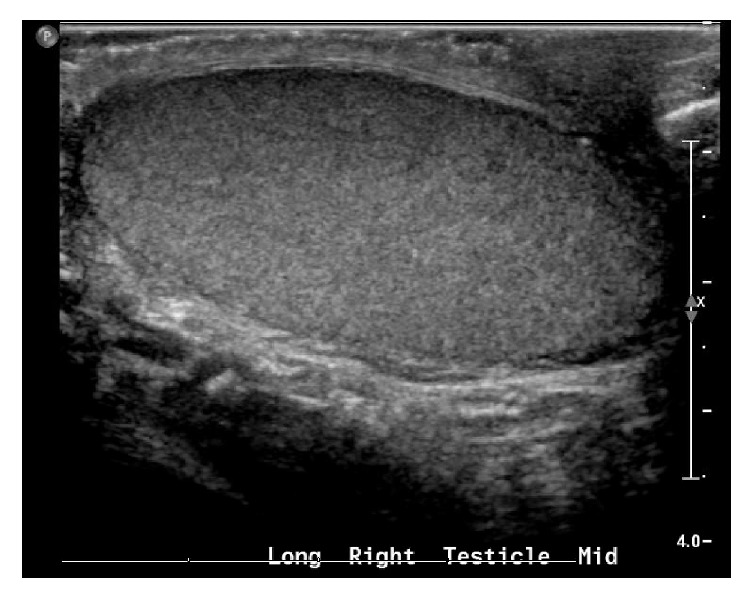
Ultrasound of normal right testicle.
